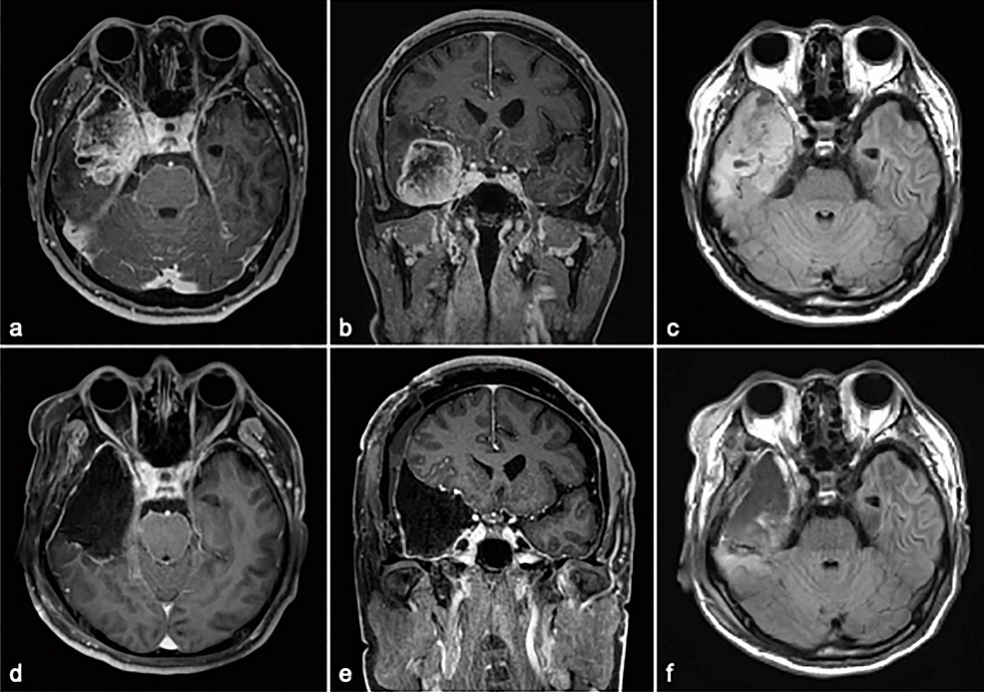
Series of MRI during the pre and postoperative course (a and b) T1-weighted imaging enhanced with gadolinium contrast showed a lesion of 5 cm in diameter with irregular margins and indistinct borders with heterogeneous contrast enhancement on axial and coronal views. (c) Peritumoral edema was identified as a hyper-intense signal on FLAIR imaging. Postoperative MR imaging presented gross total tumor removal (d and e), including the peritumoral high-intensity region on FLAIR imaging (f). FLAIR: fluid-attenuated inversion recovery
